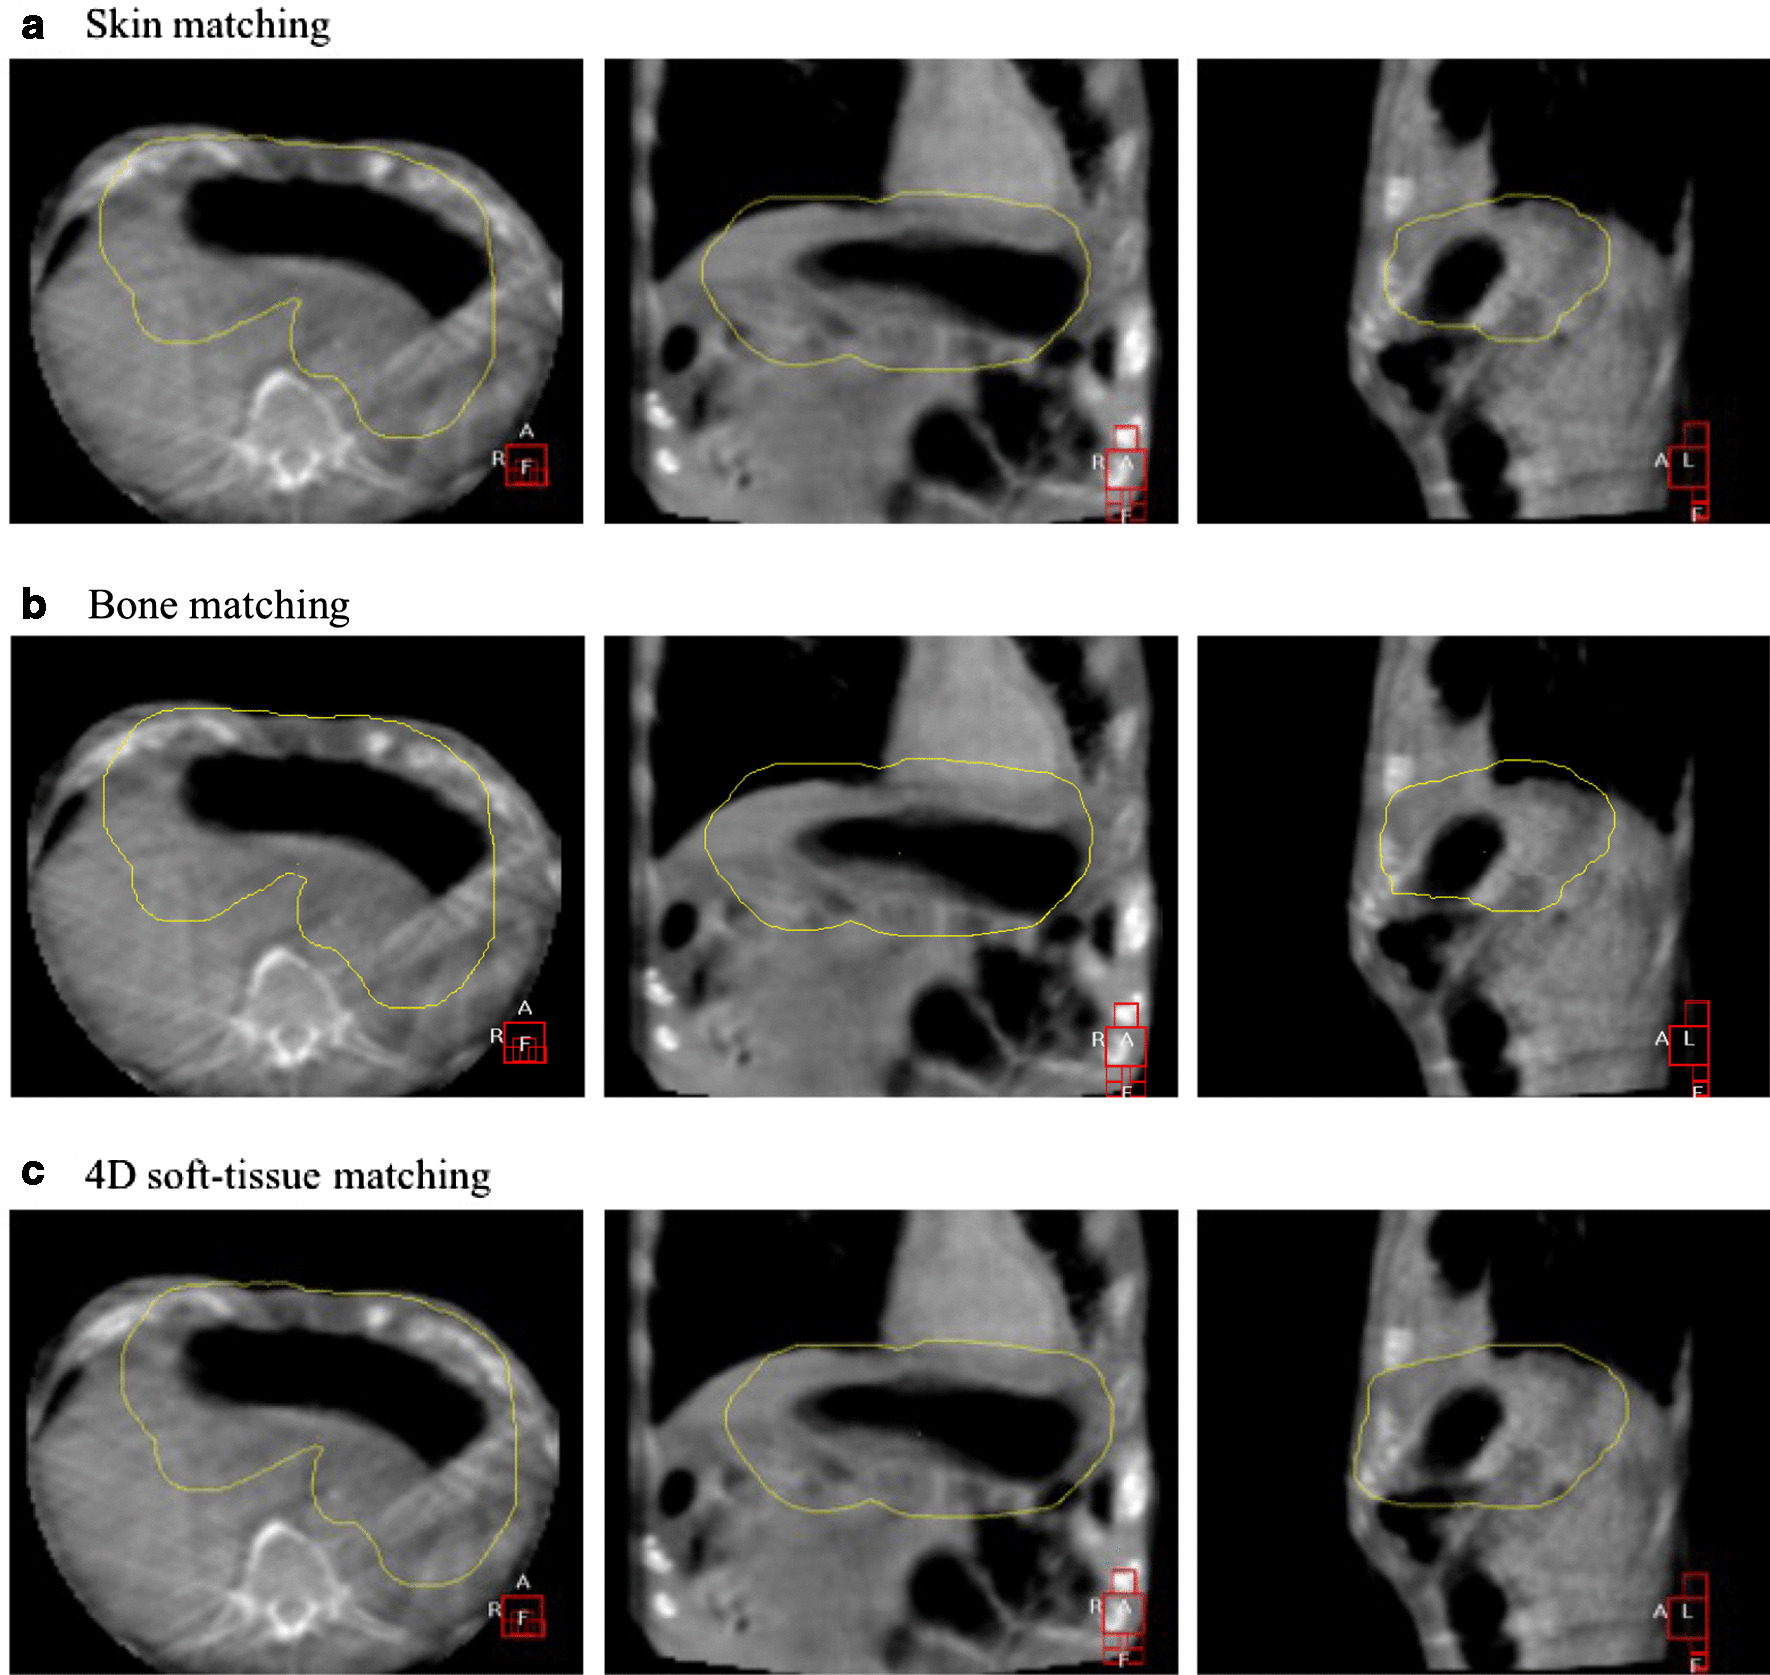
Representative images (patient number 4) of the positional discrepancy between target localization methods based on skin matching (a), bone matching (b), and 4D soft-tissue matching (c) using 4D-CBCT. A PTV structure of 15 mm (yellow) margin from the CTV defined based on the 4D-CT (CTV-4D) is displayed in the 4D-CBCT images. Target localization by skin and bone matching could not cover the entire stomach in the PTV (a, b). Target localization by 4D soft-tissue matching was able to cover the entire stomach in the PTV (c)
